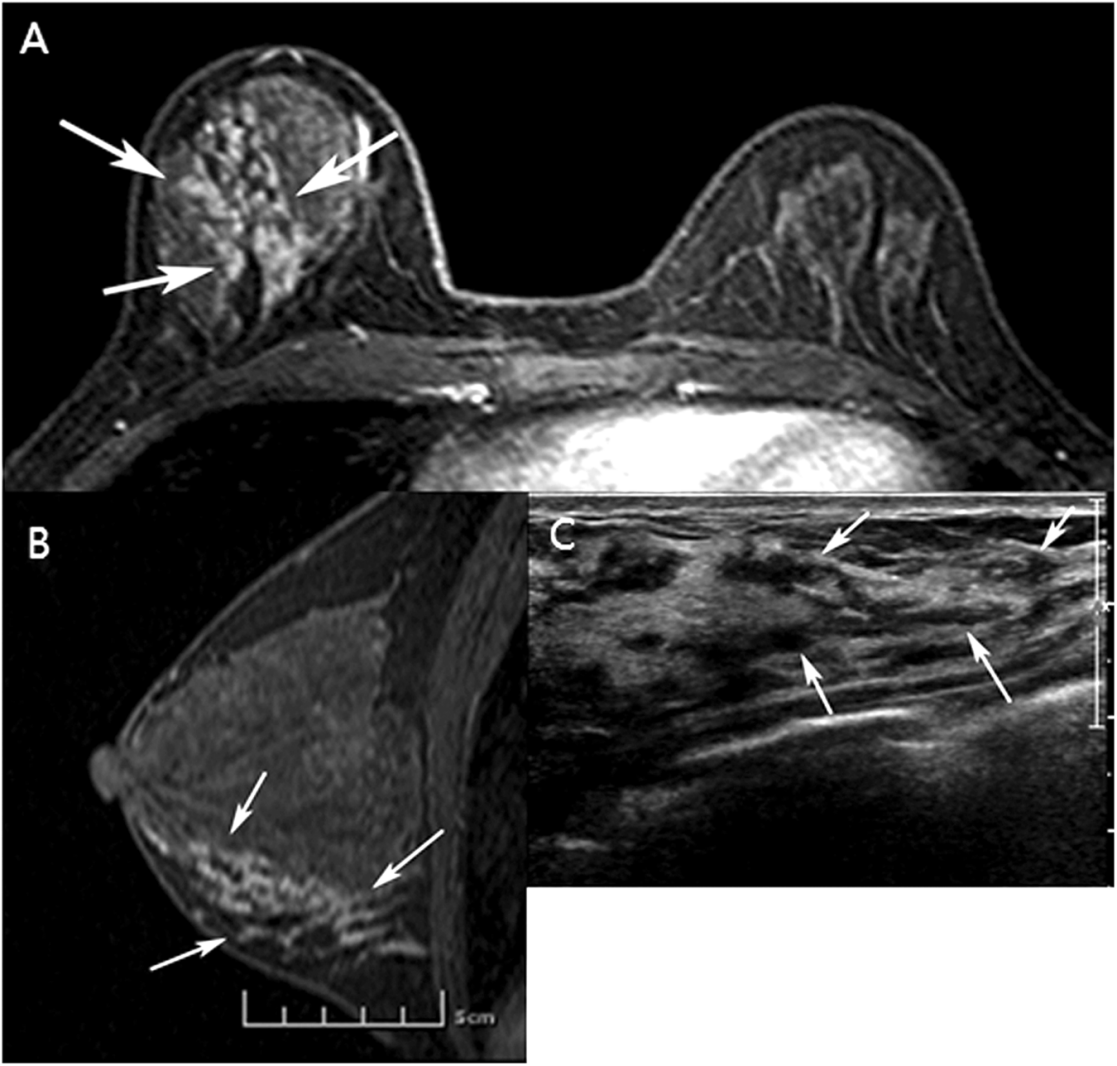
Invasive ductal carcinoma in the upper inner quadrant of the right breast of a 39-year-old woman. (A, B) Axial (A) and reformatted sagittal (B) T1-weighted magnetic resonance imaging (MRI; T1WI) with early post-gadolinium enhancement show segmental non-mass enhancement in the lower portion of the right breast (arrows). (C) Ultrasound of the non-mass lesion demonstrates a correlation with MRI findings (arrows). This non-mass lesion was confirmed as ductal carcinoma in situ via 13-gauge vacuum-assisted biopsy and surgery.
